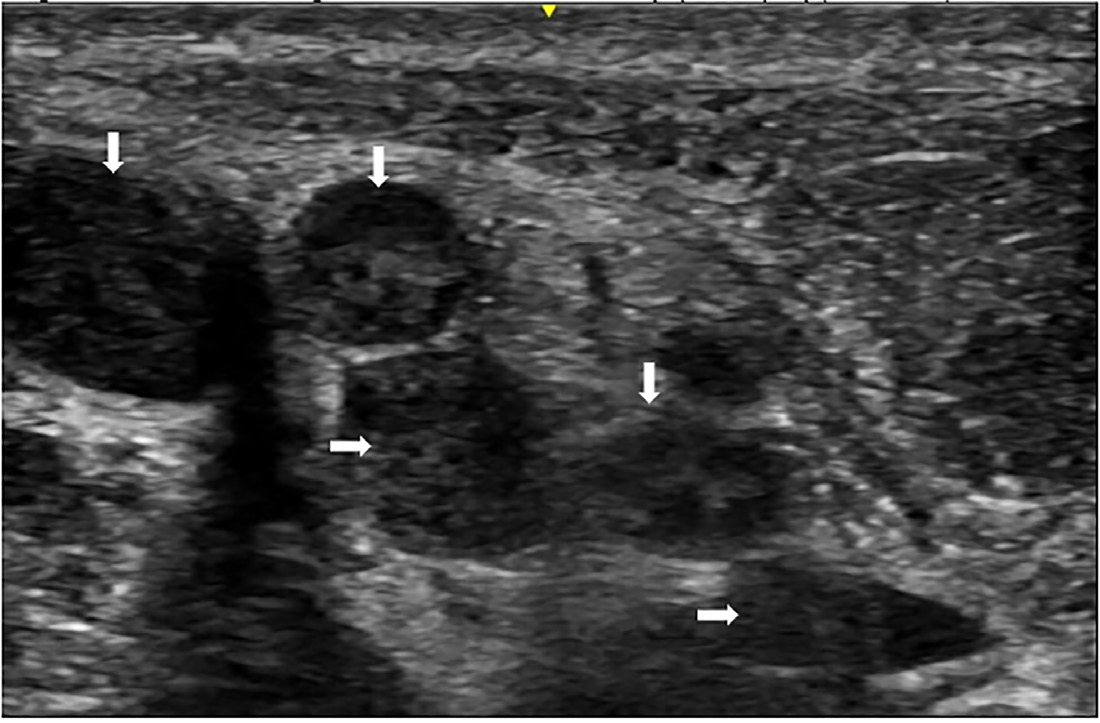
Point-of-care ultrasound images with extensive cervical reactive lymphadenopathy (white arrows).
